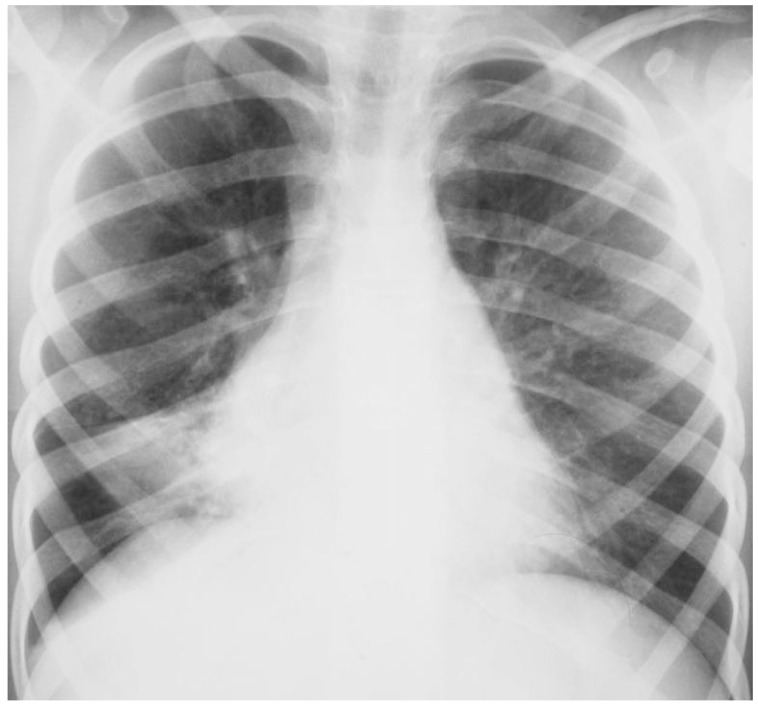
Chest radiography of a 7-year-old girl with middle lobe syndrome.
